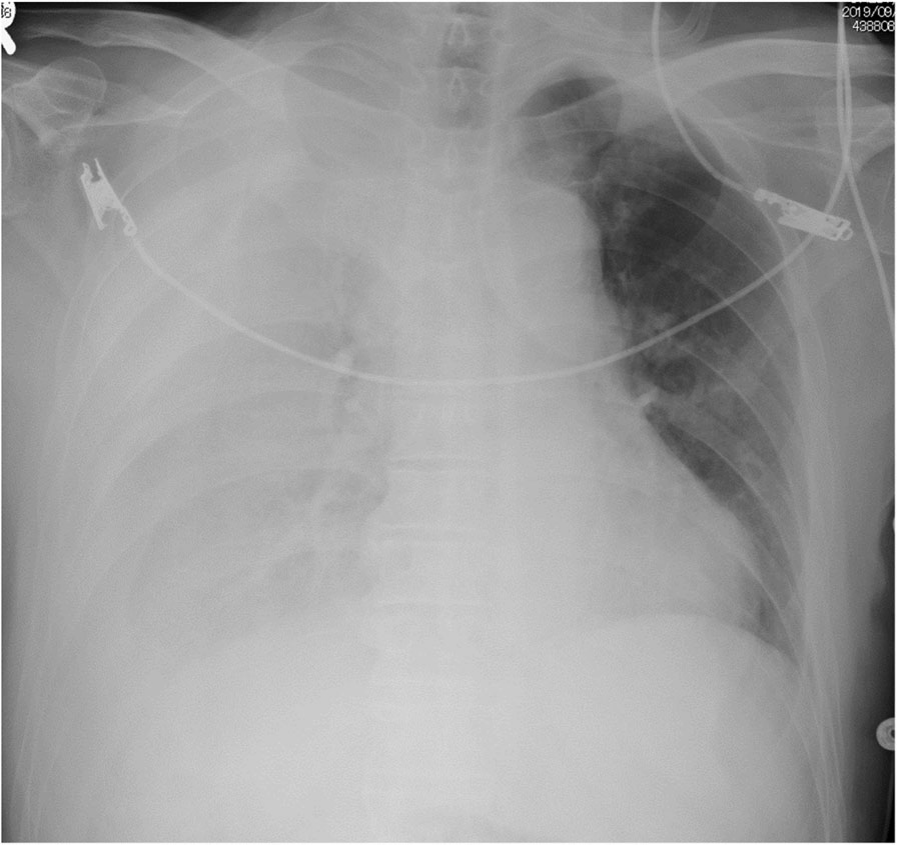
Chest X-ray showing decreased permeability of the right lung field
